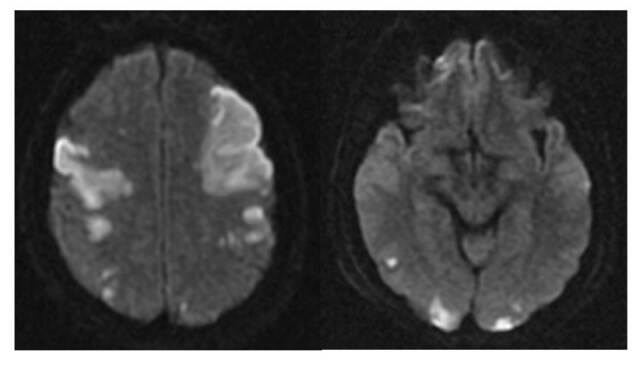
MRI (DWI) showing multiple areas of bilateral fronto-temporal, and occipital ischemia.
